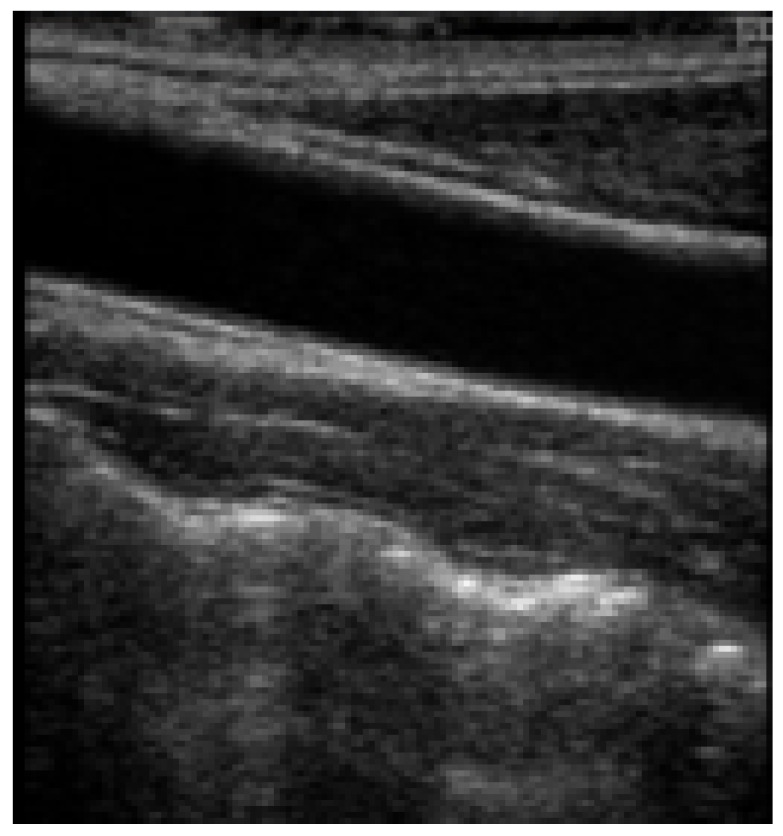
B-mode ultrasound image from publicly available CUBS dataset [31]. Accessed by CC by NC 4.0 license.
